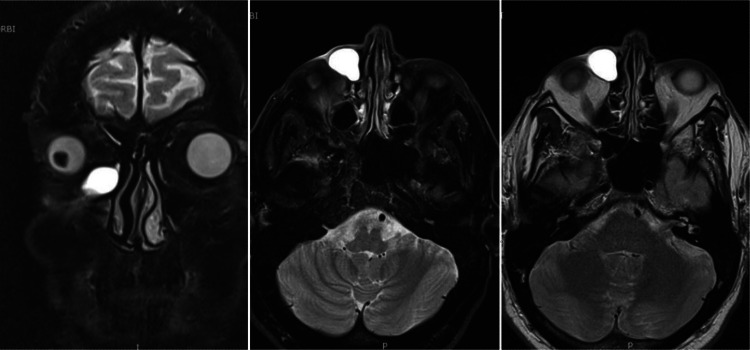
DacryoMRI of the head, T2-weighted With the help of an expert radiologist, the boundaries and dimensions of the lacrimal sac can be clearly highlighted, using simple artificial tear drops applied to the patient’s corneal surface and drained as a more physiological contrast
